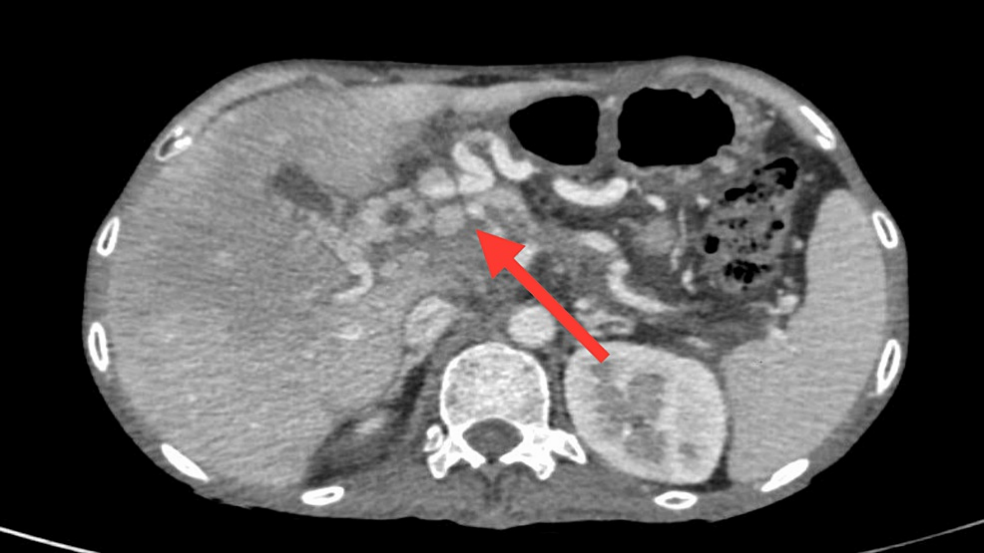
This CECT abdomen axial section shows the portal vein which is completely replaced by a tuft of dilated tortuous collaterals - cavernoma formation as shown by the red arrow. CECT: contrast-enhanced computed tomography
